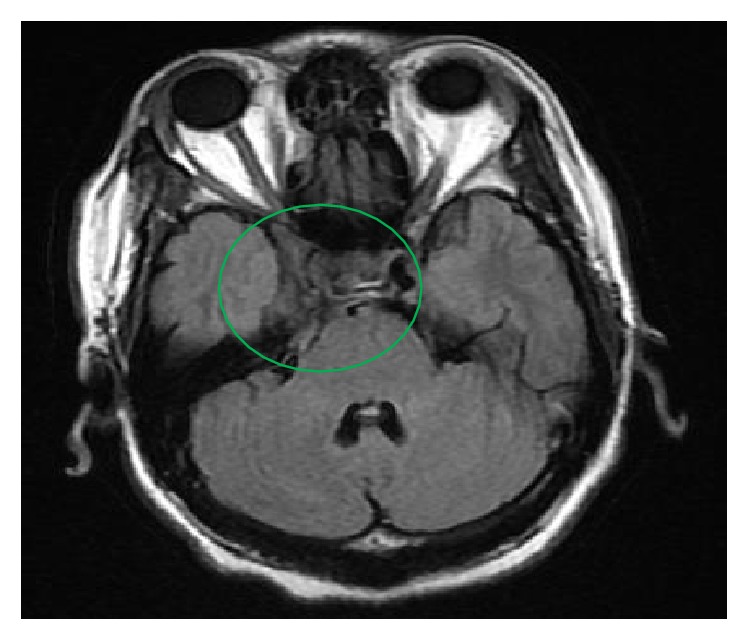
Initial cranial MRI revealing abnormal enhancement involving the right cavernous sinus area extending along the tracks of the right fifth, seventh, and eighth cranial nerves.
